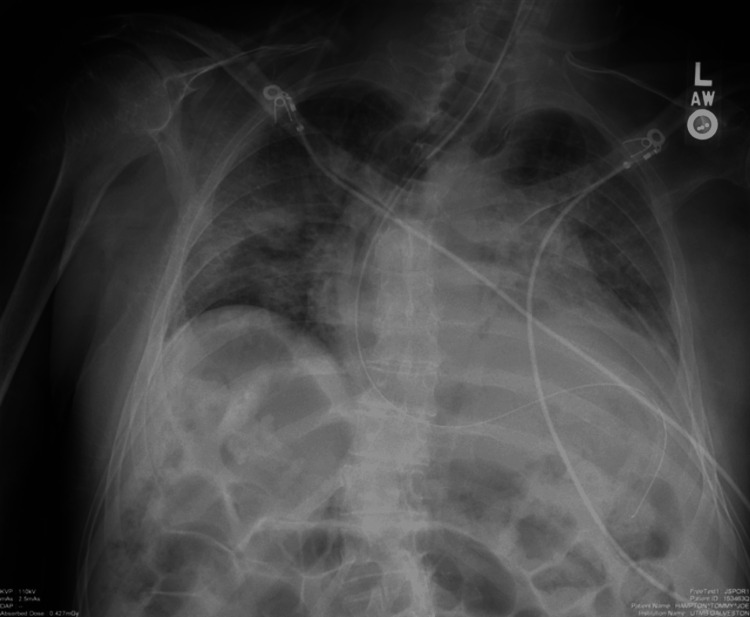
Chest X-ray after OG tube placement OG: orogastric
